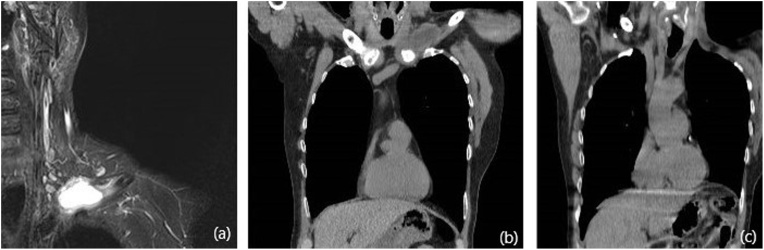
Coronal planes preoperatively in MRI (a) and CT (b) scan. Imaging one year after removal of clavicle, first rib and tumor. No evidence of disease recurrence (c).
